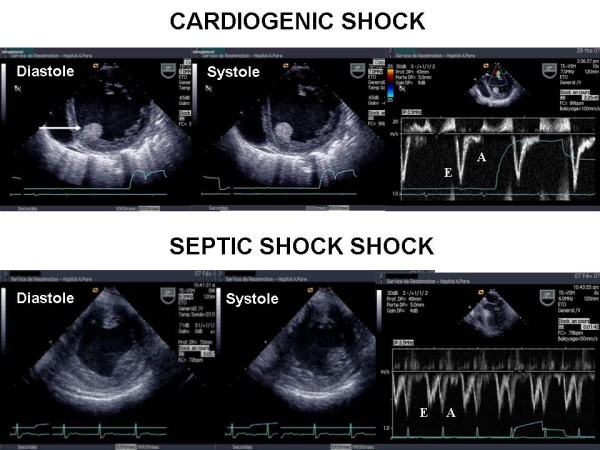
Transesophageal echocardiography in two patients--one with cardiogenic shock (above) and the other with septic shock (below). In the patient with cardiogenic shock, the left ventricular short-axis view demonstrated global hypokinesia of the left ventricle with major dilatation. Pulsed Doppler at the mitral valve demonstrated a restrictive pattern of the left ventricular inflow with a high E wave velocity and a very low A wave velocity, highly suggestive of a high LV filling pressure. Note also the thrombus in the left ventricle (arrow). In the patient with septic shock, the short-axis view also demonstrated global hypokinesia of the left ventricle, but without a major dilatation. Note also the Doppler profile at the mitral valve, highly suggestive of normal LV filling pressure.
